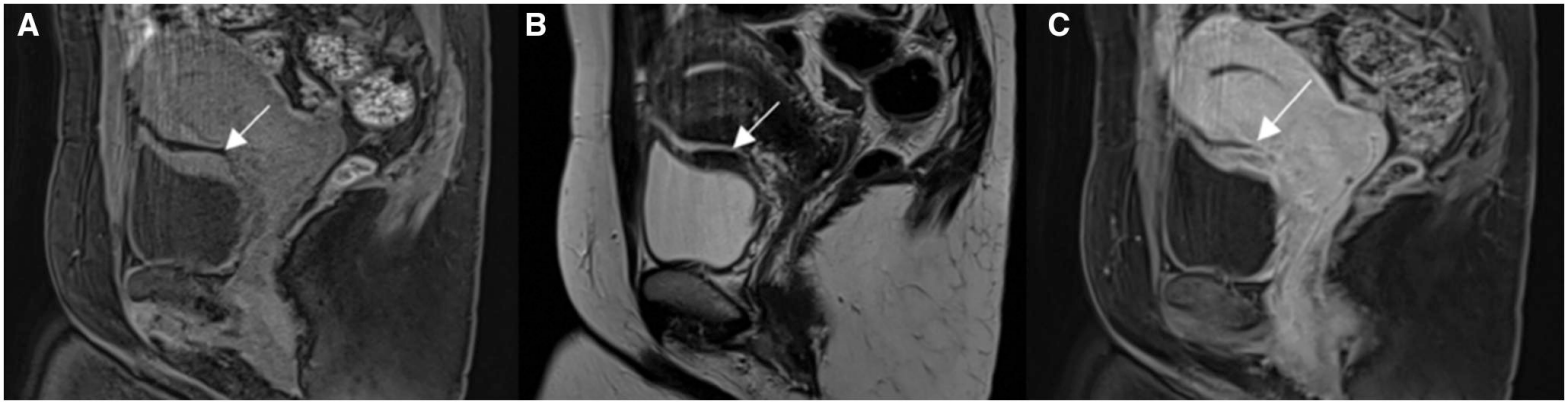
47-year-old female with biopsy-proven bladder wall endometriosis (arrow). MRI pelvis demonstrates thickening of the bladder dome with (A) intermediate signal on pre-contrast fat saturated T1weighted imaging, (B) low signal on T2 weighted imaging and (C) inhomogeneous enhancement post-contrast administration.
